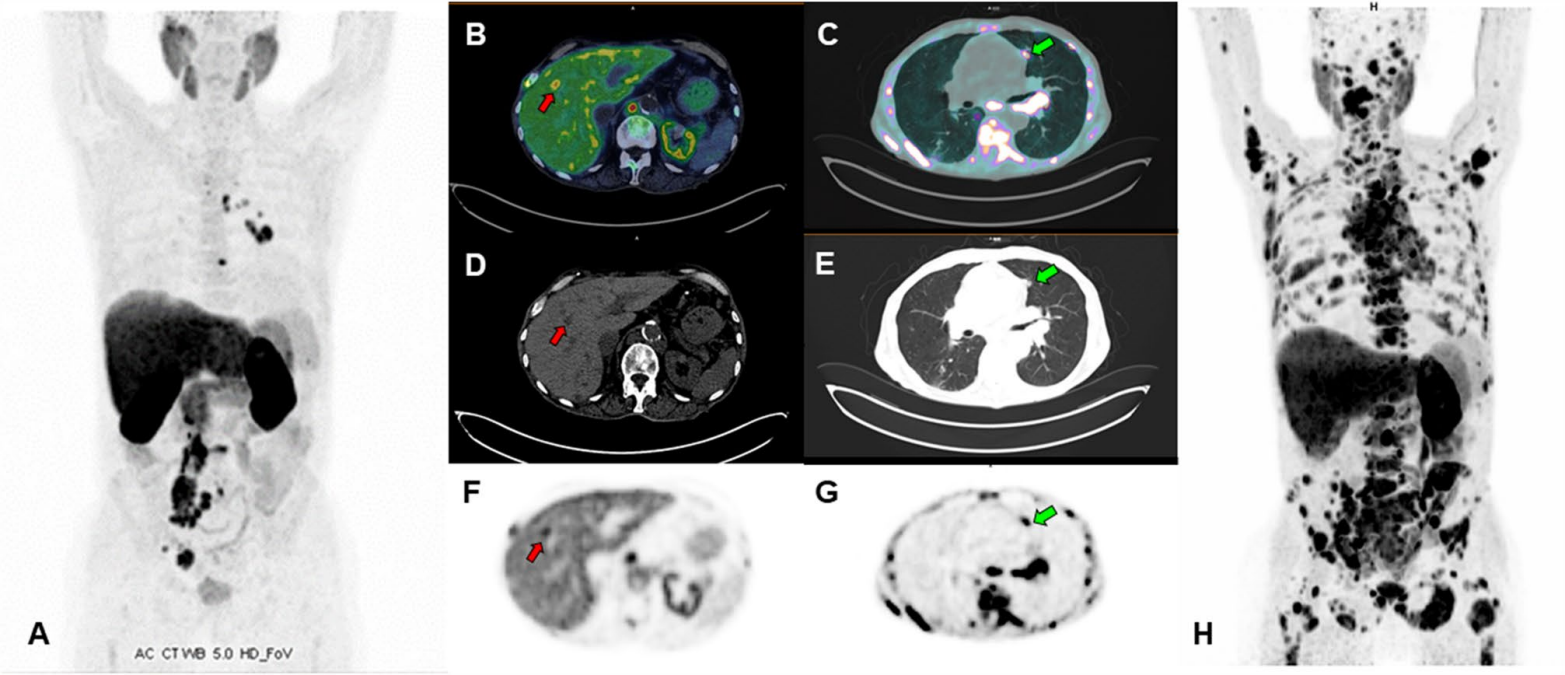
Baseline [18F]F-choline PET/CT scan (A maximum intensity projection) showed several skeletal and lymph nodes PCa metastasis uptake. Restaging [18F]F-choline PET/CT scan (B,C transaxial fused images; D,E CT images; F,G emissive images; H: maximum intensity projection), performed 12 months later, after starting treatment with metronomic cyclophosphamide, showed a multiple district disease progression, with lesions involving lymph nodes, bone, liver (red arrows, SUVmax 15) and both lungs (green arrows, SUVmax 5.1)
